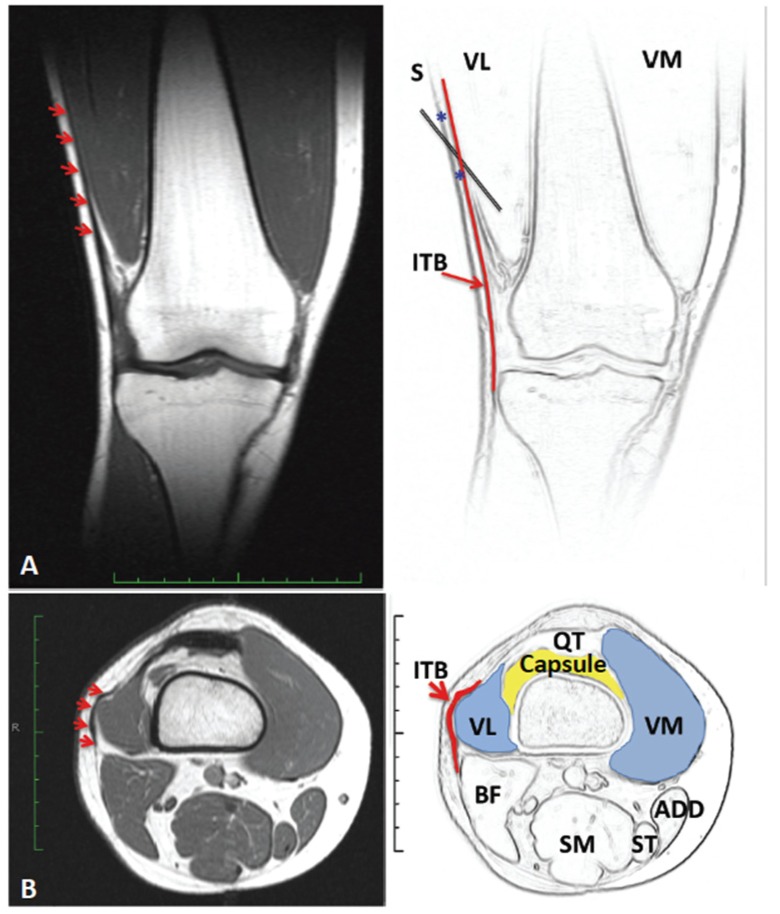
Coronal plane MRI (A) to highlight the relationship of the Iliotibial band to the Vastus Lateralis muscle (red arrows). Axial plane MRI to highlight the anatomy at the level of the superior pole of the patella (B). Note the proximity of the lateral joint capsule (supra-patella pouch) to the distal end of the VL and the overlying ITB to the VL muscle (red arrows); Legend: VM = vastus medialis, VL = Vastus lateralis, ITB = Iliotibial band, BF = Biceps femoris, SM = Semimebranosus, ST = Semitendinosus, ADD = Adductors, QT = Quadriceps tendon.
